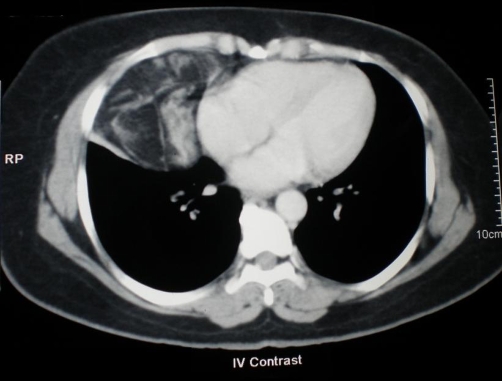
Lesion in the anterior mediastinum which had low CT attenuation values suggestive of a lipoma.
